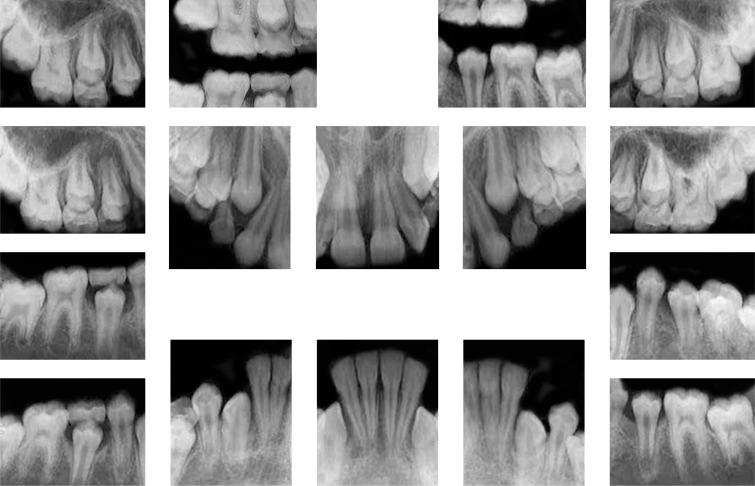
Initial periapical radiographs.
